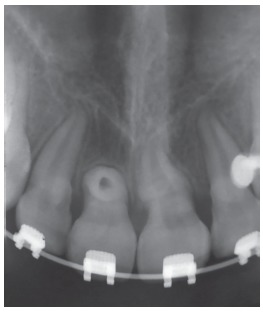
Post-alignment IOPA x-ray showing the characteristic “bull’s-eye” appearance of the dilacerated root.
